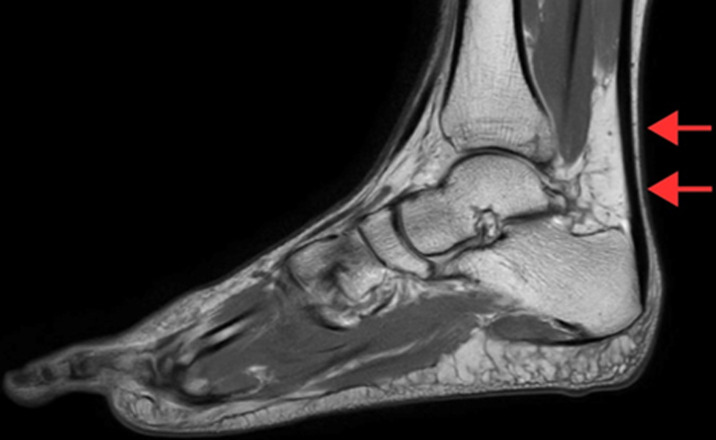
sagittal T2-weighted MRI highlighting Kager’s fat pad syndrome (arrows) and associated retrocalcaneal bursitis
